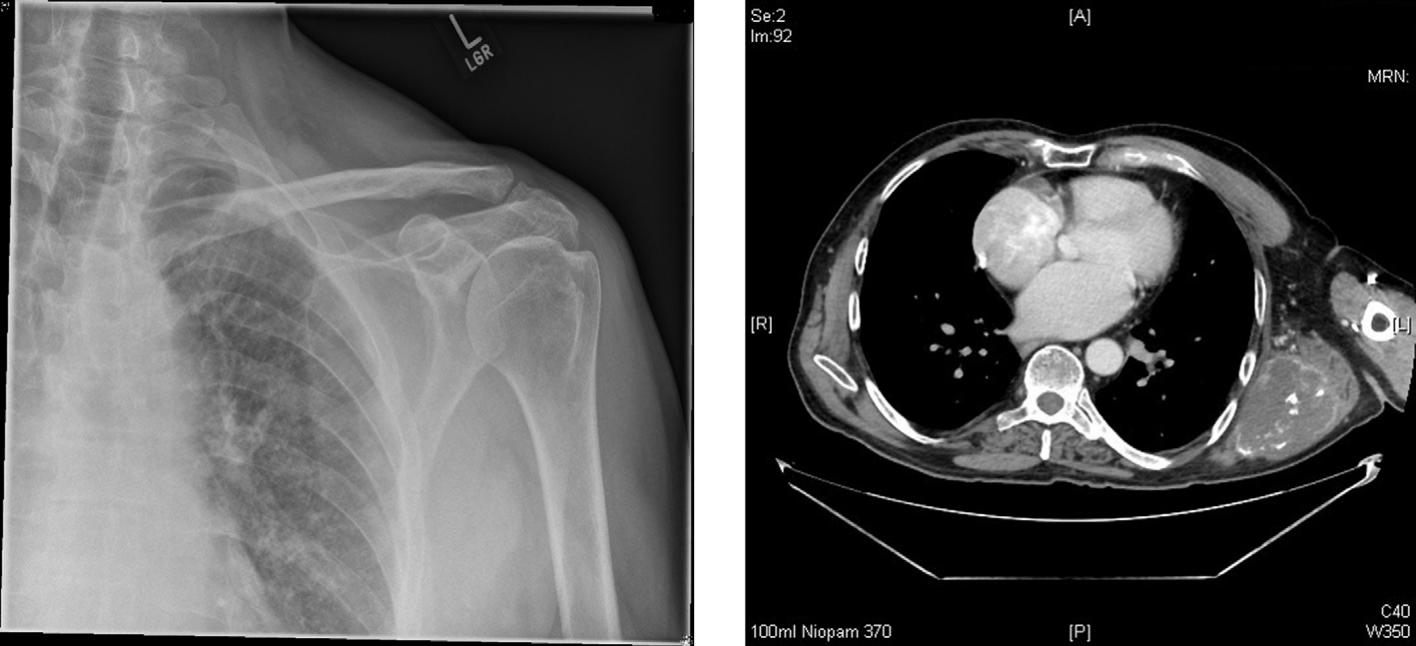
Plain radiograph of left shoulder girdle only showing some soft tissue swelling around the lateral border of the scapula whereas the CT scan demonstrates a destructive lesion consistent with chondrosarcoma.
